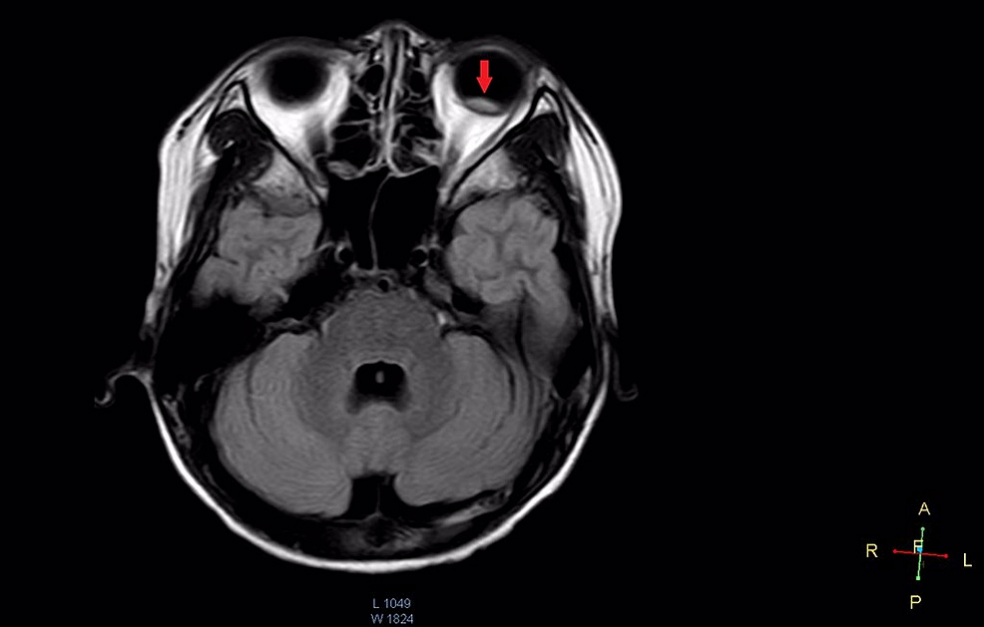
Magnetic resonance imaging of brain and orbit showing an elliptical lesion at the posterior aspect of the left globe with diffuse thickening of the left optic nerve in the second case.
